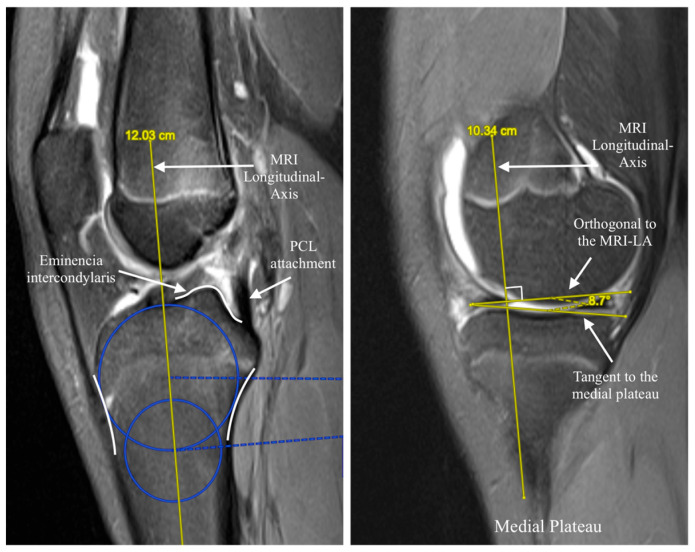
Sagittal MRI slice of the knee demonstrating the 2-circle method for measuring the medial posterior tibial slope (MPTS). (Left): Defining the MRI Longitudinal Axis of the tibia (MRI-LA). (Right): Measuring the angle between the tangent to the medial plateau and the line orthogonal to the MRI-Longitudinal Axis.
